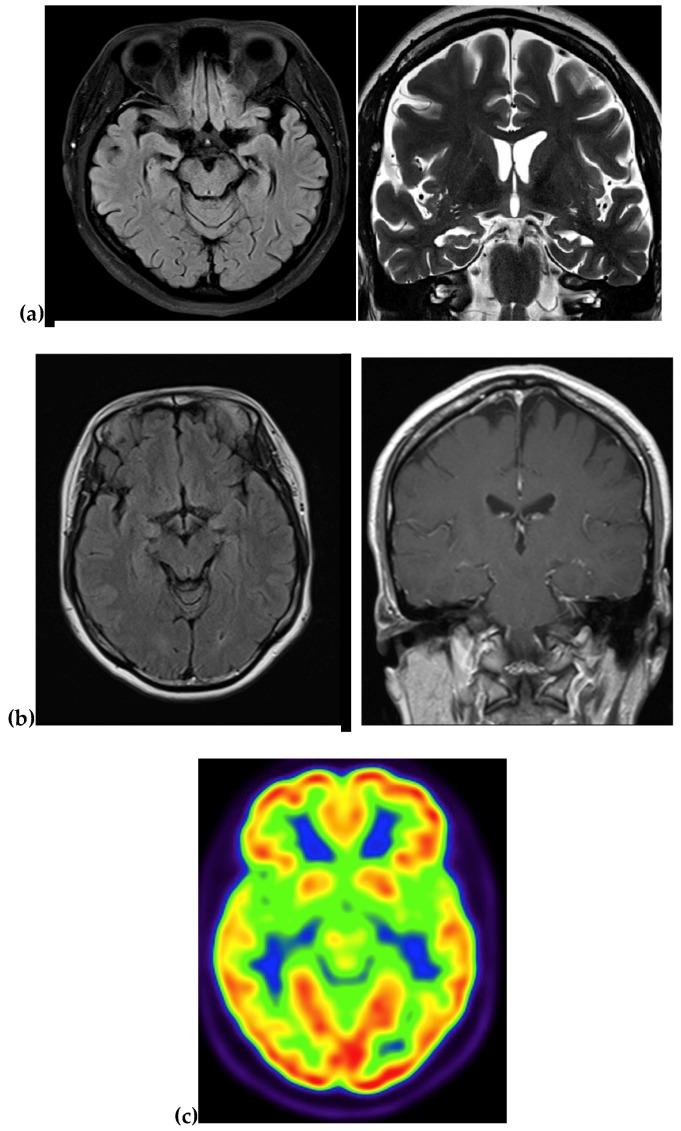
(a) MRI data of proband patient of Family 1. Atrophy could be seen in the right temporal and parietal regions. A vascular abnormality also appeared in the left frontal area. (b) MRI data of proband patient of Family 2. (c) PET data from Family 2.
